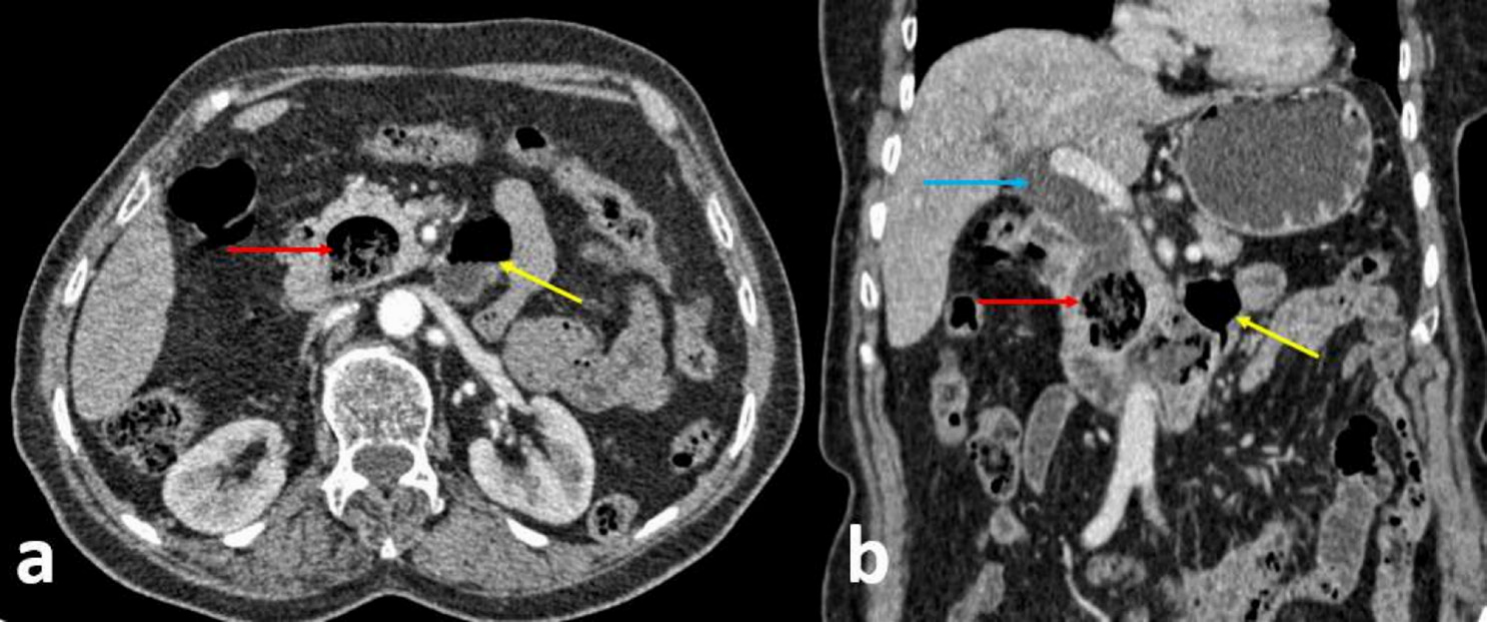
Computed tomography scan of the abdomen with contrast on axial plan (a) and coronal reconstruction (b) showing a 23 mm periampullary duodenal diverticulum in the medial wall of the second portion of the duodenum with containing fluid and air (red arrow) strictly adjacent to the distal portion of the common bile duct (measuring 12 mm in calibre) and causing the dilatation of the bile ducts (blue arrow). Next to the third portion of the duodenum, there was a second diverticulum measuring about 25×21 mm (yellow arrow).
